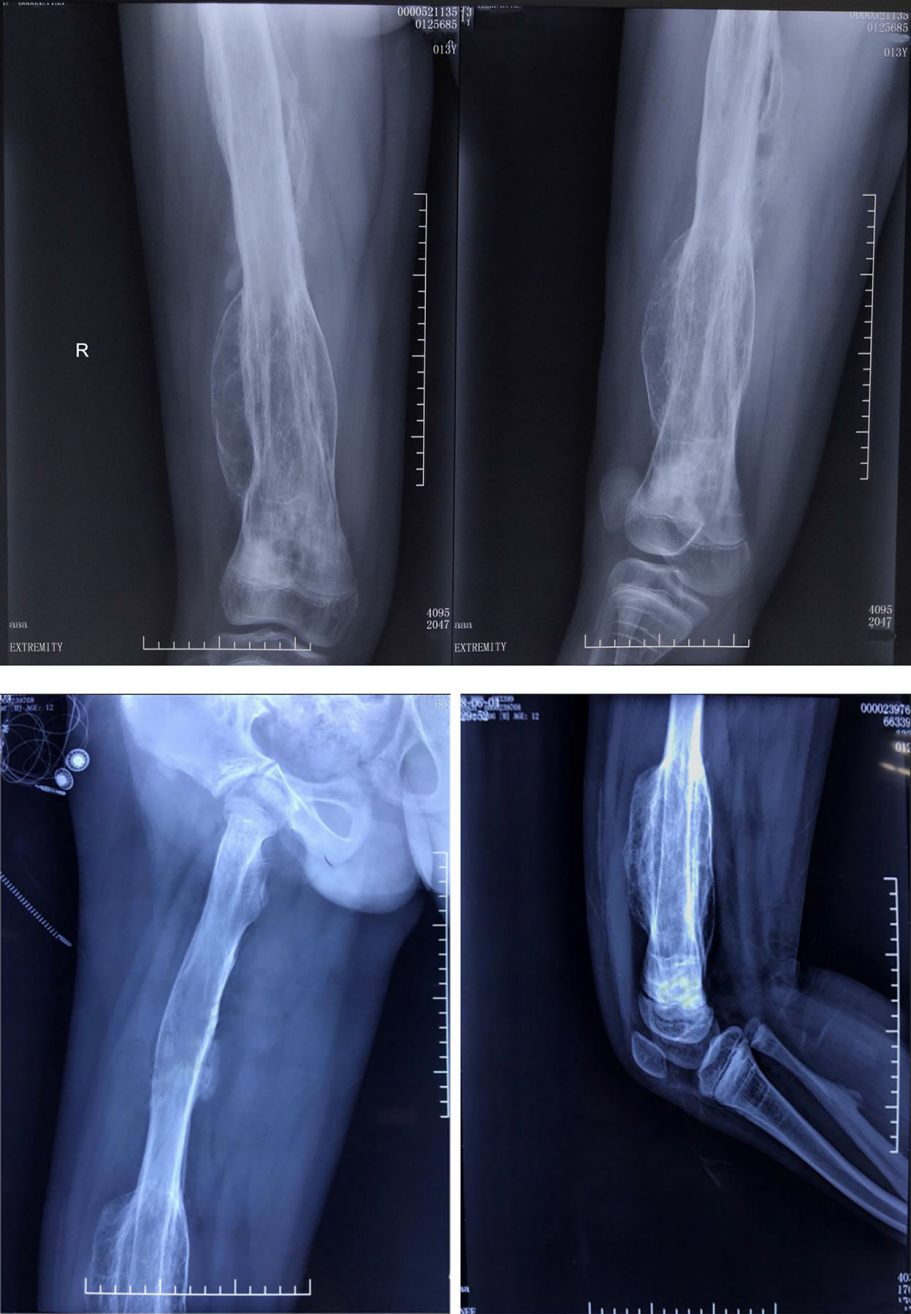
X-ray of the right femur indicated inhomogeneous bone density losses of the right femur, cortical thinning, transverse sclerotic lines at the right distal femur and interface of the epiphysis and metaphysis of the proximal tibia, and excessive callus formation at the cortical margins.
